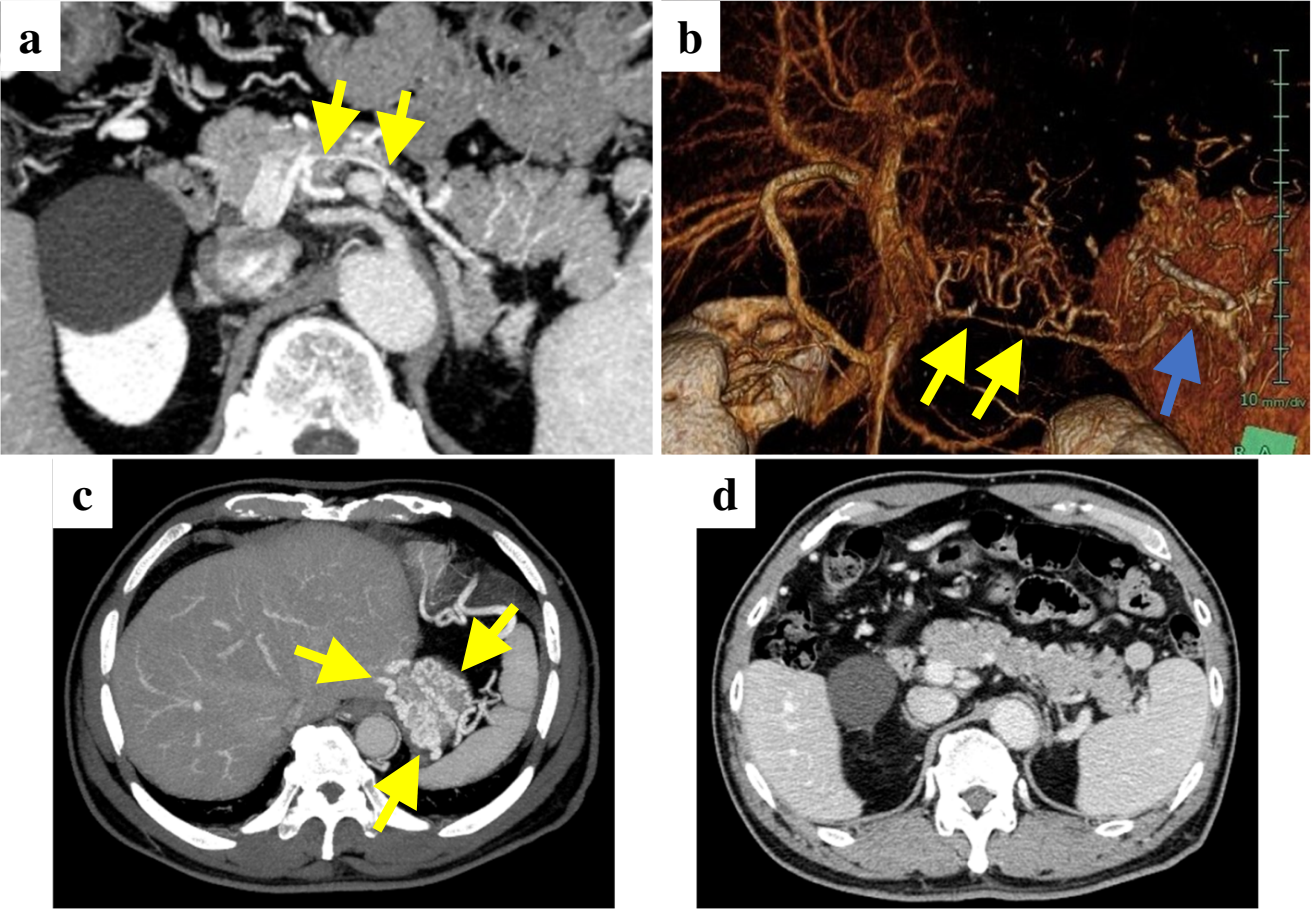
Contrast-enhanced abdominal computed tomography findings. a, b Stenosis (2 mm in diameter, yellow arrows) of almost the entire length of the splenic vein, with post-stenosis dilatation at the splenic hilum (10 mm in diameter, blue arrows). c Development of gastric fundal varices and short gastric veins. d No inflammatory changes around the pancreas or pancreatic neoplasm were observed. a, c, d Axial views. b Blood vessel construction image
